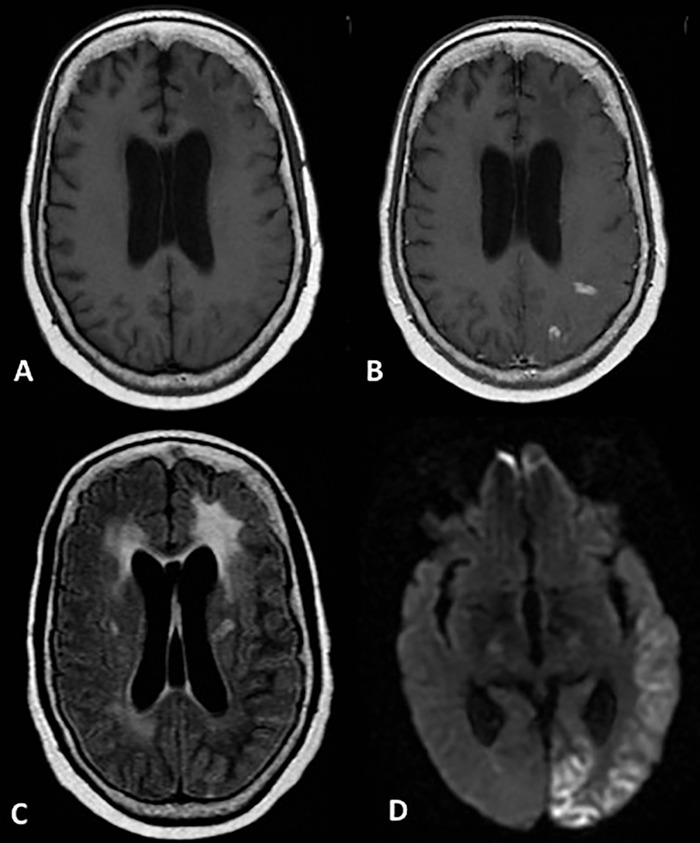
Follow-up magnetic resonance imaging 1 week later. Axial T1-weighted images through the level of the cerebral hemispheres before (A) and after (B) the administration of gadolinium contrast demonstrate few remaining foci of cortical enhancement involving the left posterior parietal and occipital lobes, with corresponding mild residual T2/fluid attenuated inversion recovery signal along the left occipital lobe (C) and persistent cortical restricted diffusion involving the left posterior parietal and occipital lobes on diffusion weighted imaging (D).
